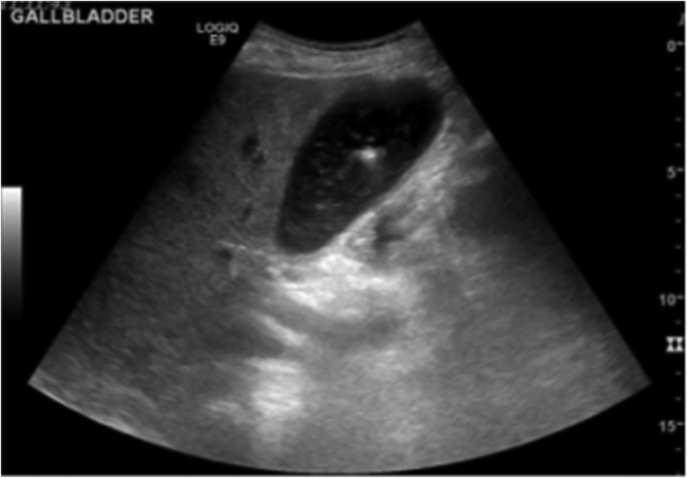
Ultrasound of the gallbladder identified thickening of the gallbladder and a small stone.
